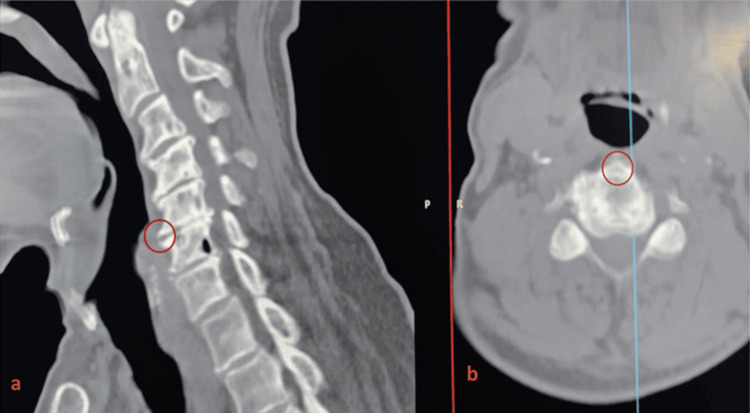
Computed tomography scans disclosing the pre-operative radiological bony detail examination for patients who showed cervical disc disease manifestations (a) Sagittal and (b) axial. The red circle indicates anterior osteophyte.
